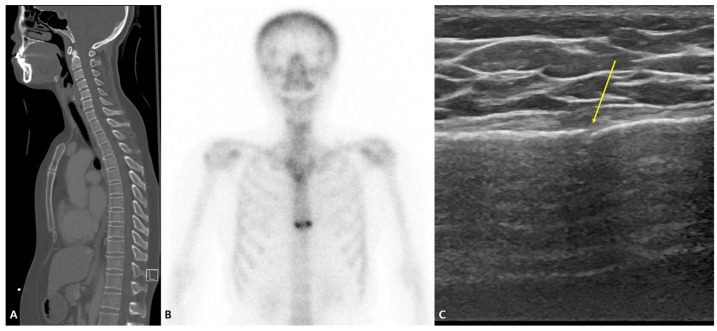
Three images of a case (number 22 in Table 3) with the (A) initial chest CT scan (−), (B) bone scan (+), and (C) ultrasound (+). Loss of continuity in the sternal cortex (arrow).
